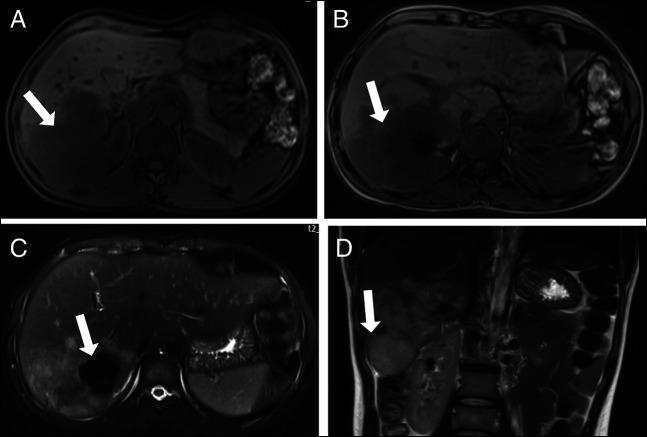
Contrast-enhanced CT scan of the abdomen. (A) Axial section showing a large CNSET with central calcification (black arrow). (B) Axial section showing a exophytic component of the tumor (black arrow). (C) Axial section showing the involvement of a major portion of the right hepatic lobe (black arrow). (D) Axial section showing tumor invasion into the right posterior portal vein (black arrow). CNSET, calcifying nested stromal-epithelial tumor; CT, computed tomography.
